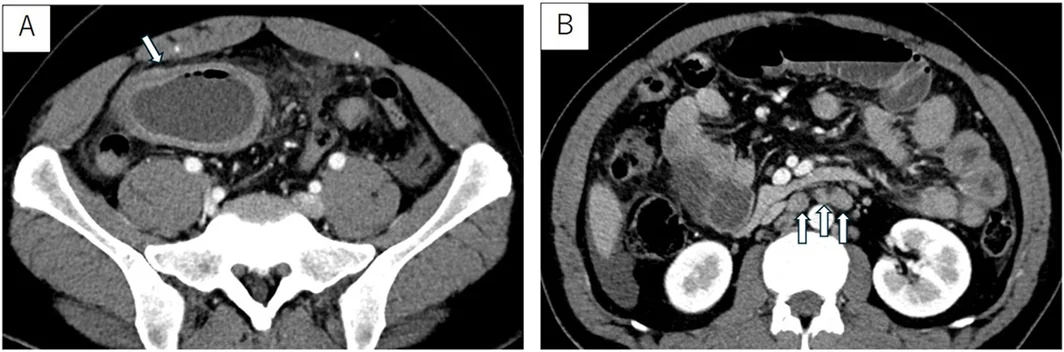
Contrast‐enhanced abdominal computed tomography (CT) performed at the time of admission revealed continuous dilatation of the small intestine, symmetric wall thickening, increased surrounding fat density, and enlarged lymph nodes (A). Multiple enlarged lymph nodes measuring more than 1 cm were also seen in the abdominal para‐aortic region (B). Arrows indicate the thickened small intestinal wall (A) and enlarged para‐aortic lymph nodes (B).
